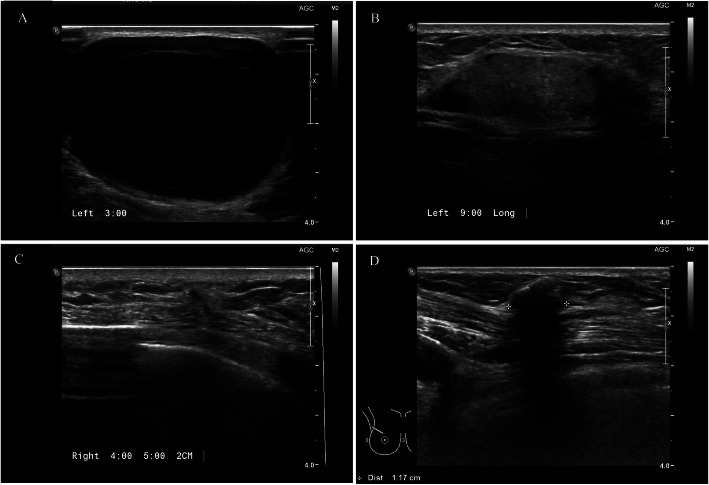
Ultrasonographic findings of fat necrosis after breast reconstruction. a, b A simple or fat-containing complex cyst is a typical ultrasonographic finding of fat necrosis. c, d Sometimes the fat necrosis mimics tumor recurrence in ultrasonography as a hypoechoic nodule with irregular margins. In these cases, pathologic confirmation is necessary to distinguish it from tumor recurrence
